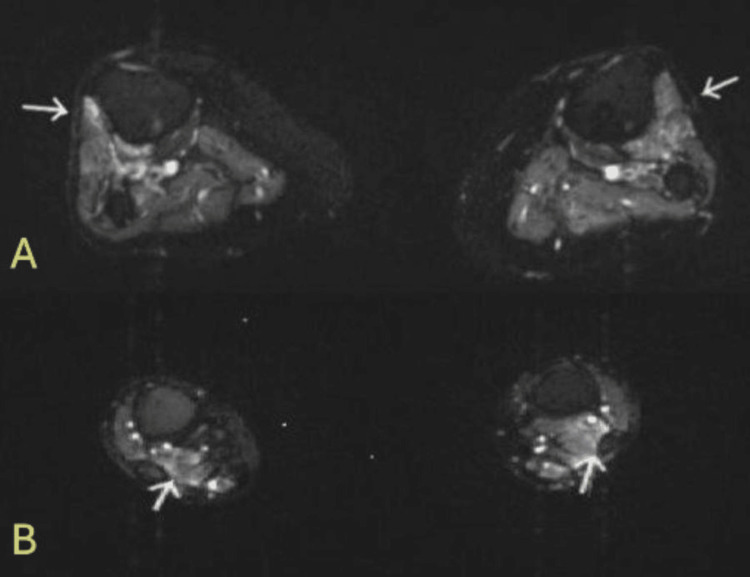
Bilateral MRI tibia and fibula without contrast, T2-weighted axial images A: arrows point to fluid-sensitive enhancement around the anterior tibialis muscles bilaterally. B: arrows point to fluid-sensitive enhancement around the flexor hallucis longus muscles bilaterally.
